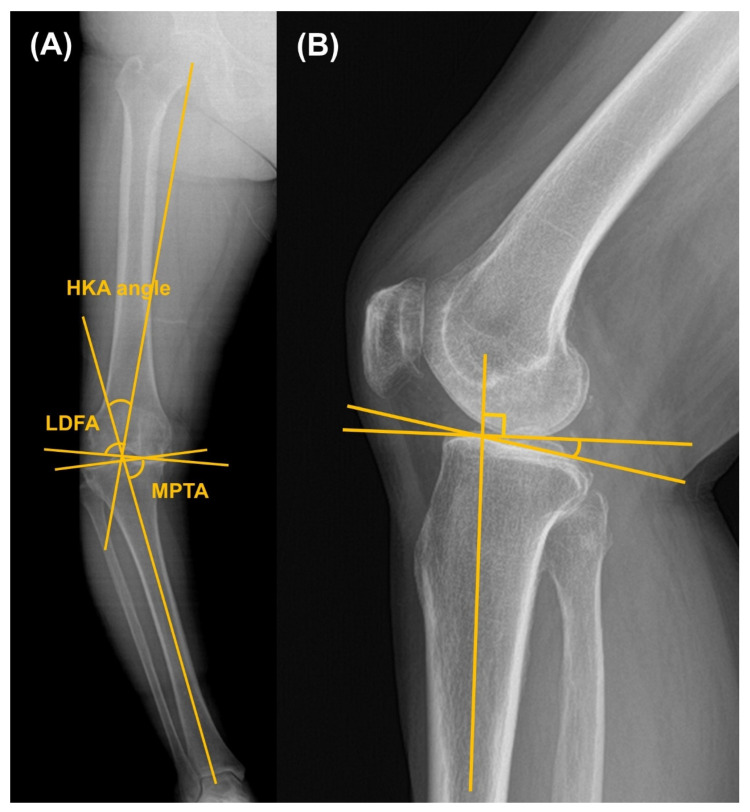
Measurement of (A) hip-knee ankle (HKA) angle, lateral distal femoral angle (LDFA) and medial proximal tibial angle (MPTA) on whole-leg standing radiographs and (B) tibial slope on knee lateral view.
